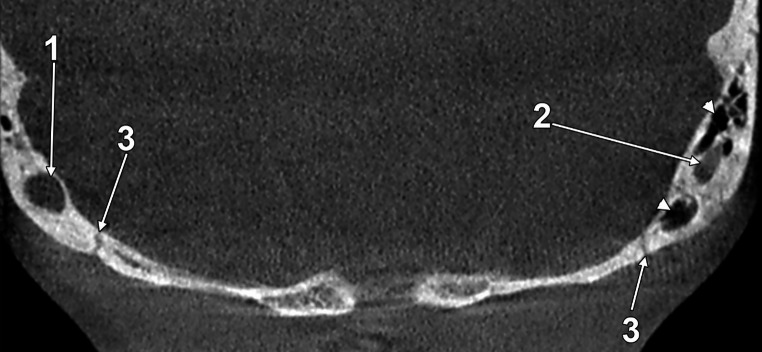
Coronal CBCT slice, anterior view. Original evidence. On the right side, a 6.08 mm-calibre mastoid canal is found (1). On the left side, a mastoid canal (2) of 3.89 mm courses among mastoid air cells (arrowheads). The occipitomastoid sutures are indicated bilaterally (3)
